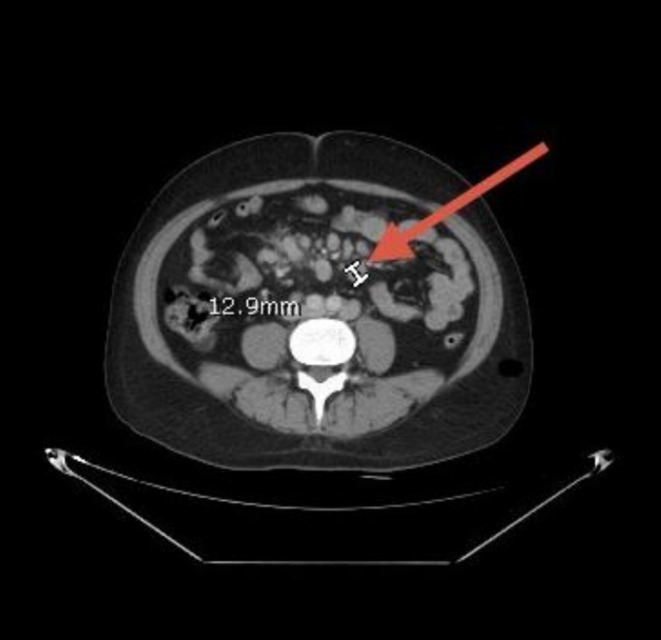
Mesenteric lymphadenopathy. Contrast‐enhanced CT of the abdomen showing enlarged mesenteric lymph nodes, reflecting intra‐abdominal nodal involvement.
